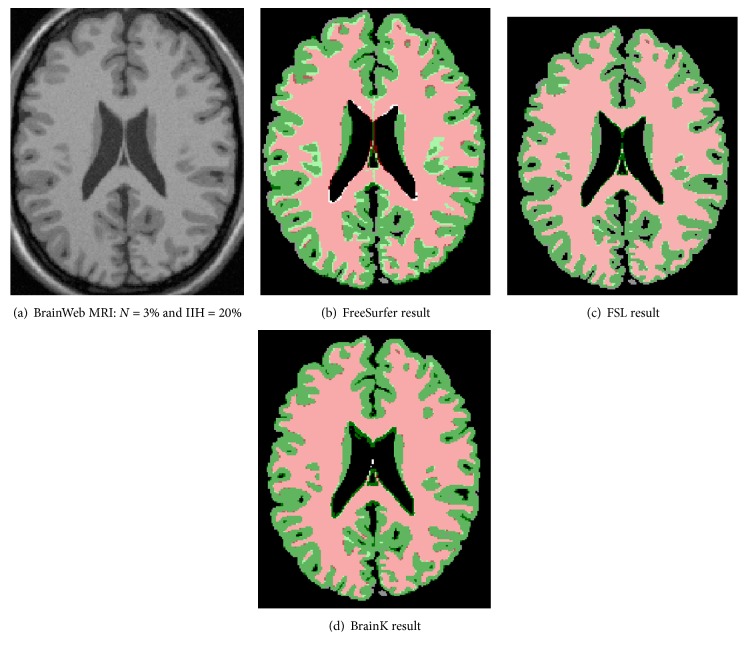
BrainK segmentation on the BrainWeb MRI compared to FreeSurfer and FSL.
